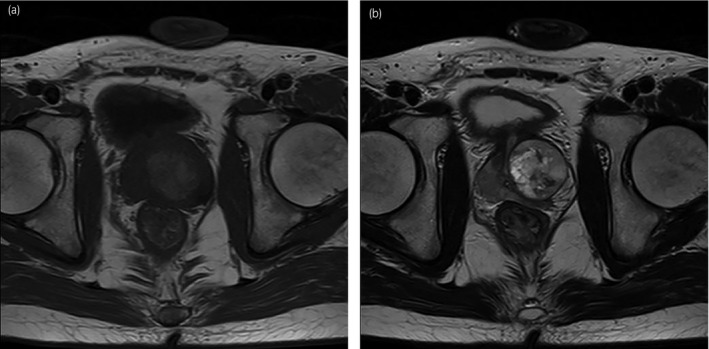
Magnetic resonance imaging (a: T1‐weighted image, b: T2‐weighted image) revealed a 4.6‐cm mass with heterogeneous high signal intensity.
